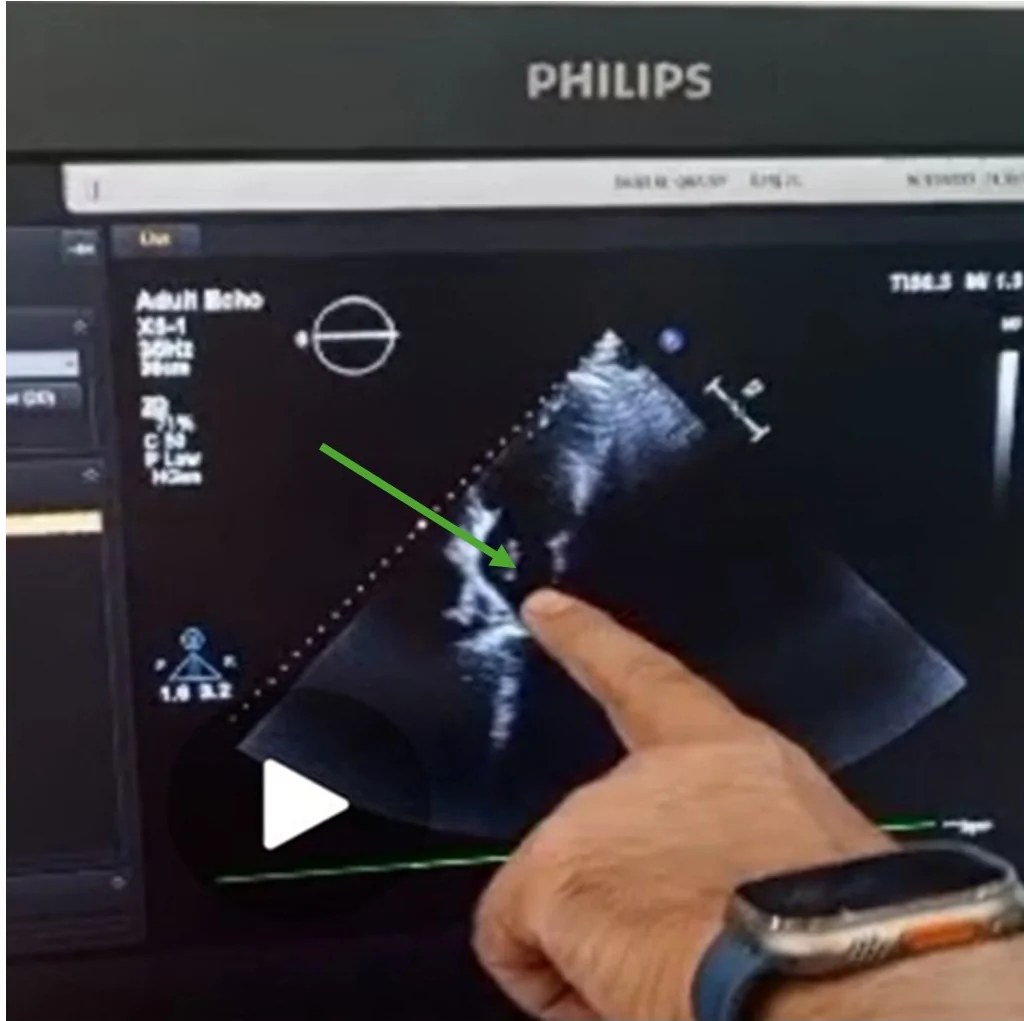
Transthoracic echocardiography showing a left atrial hydatid cyst located posterior to the heart with daughter cysts (colored arrow).
